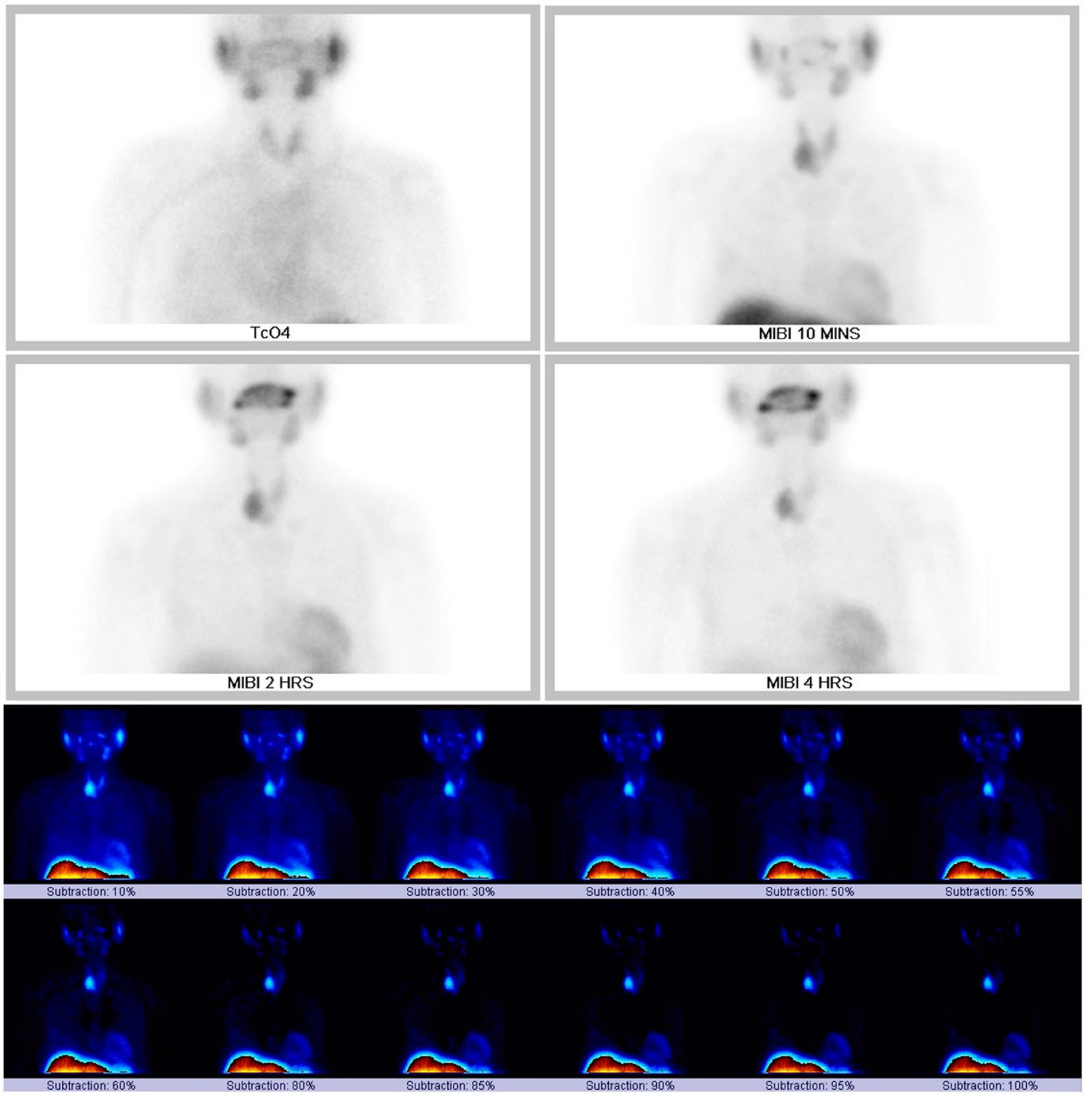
Parathyroid scintigraphy showed focal uptake of ^99m^Tc-sestamibi in the inferior pole of the right thyroid lobe on initial (10 minutes), delayed (2 and 4 hours), and subtraction images
